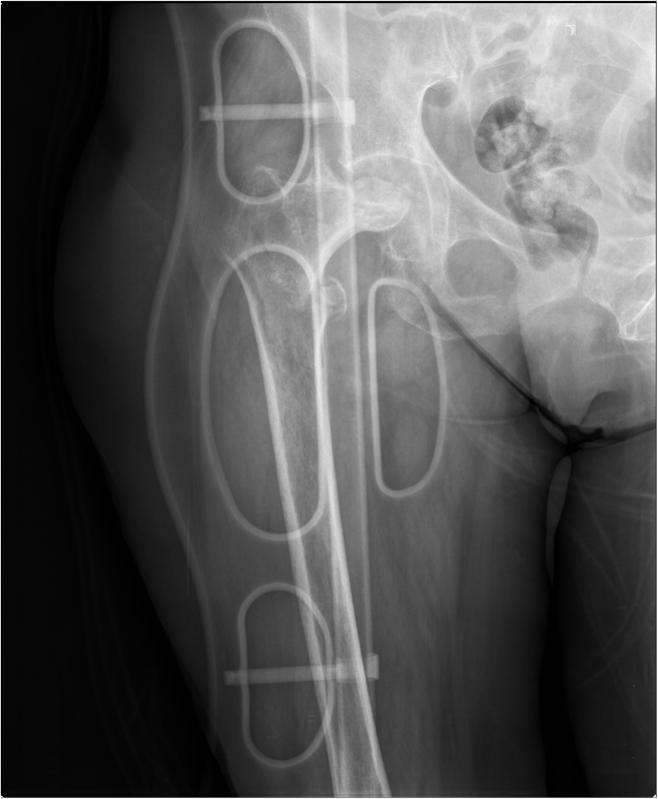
Emergency X-ray of her right hip showing a simple intertrochanteric fracture associated with femoral head stage VI avascular necrosis, with the femoral head impacted in the acetabular rim.
